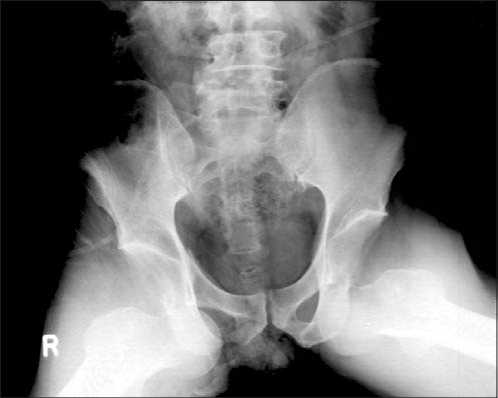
Radiograph showed bilateral anterior dislocation of both hip joints and disruption of the axial alignment at L4/5.
